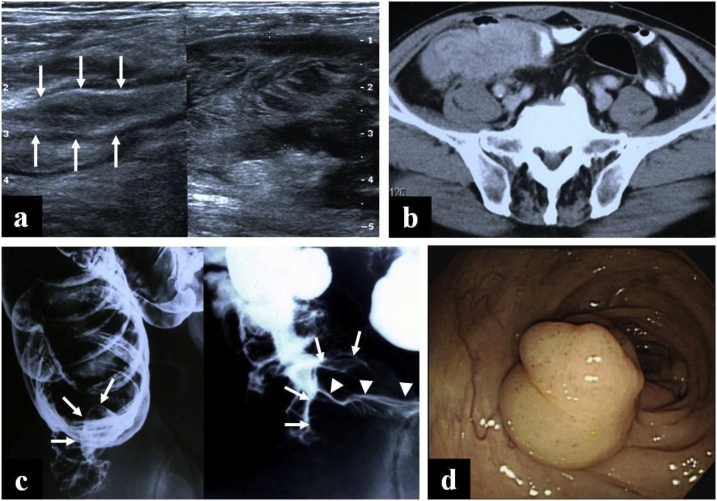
a. Ultrasonograms show intact mucosa and thickened submucosal layer with short segment intussusception (arrow) of the terminal ileum. b. Contrast enhanced CT scans represent homogeneous mural attenuation with mild pericolic infiltration. Note the short intussusception of terminal ileum. c. Colon contrast study: Short intussuscepted segment (arrow) of the terminal ileum was visualized in the cecum. Terminal ileum shows relatively intact mucosa (arrow head) and thickened fold. d. Colonoscopy shows a prominent protrusion of ileocecal valve.
